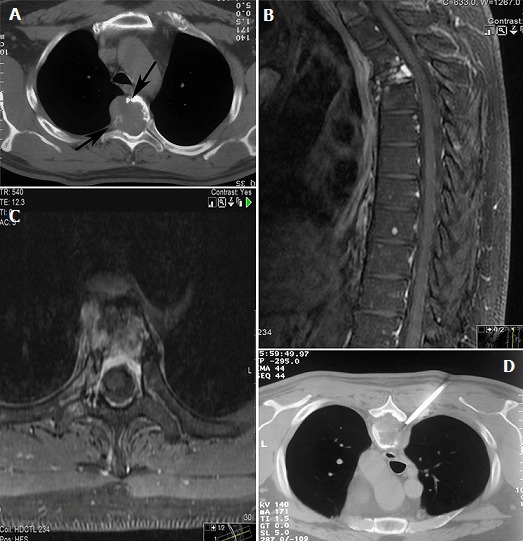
(A) CT scan depicts an expansile lytic lesion in T6 vertebra that destroys the cortex of the vertebral body (arrows), Sagittal; (B) and axial; (C) fat-saturated (FS) T1-weighted MRI with intravenous gadolinium contrast demonstrates compression fracture of vertebral body and the extent of disease in the marrow cavity, soft tissues and epidural space; (D) computed tomography guided needle biopsy
